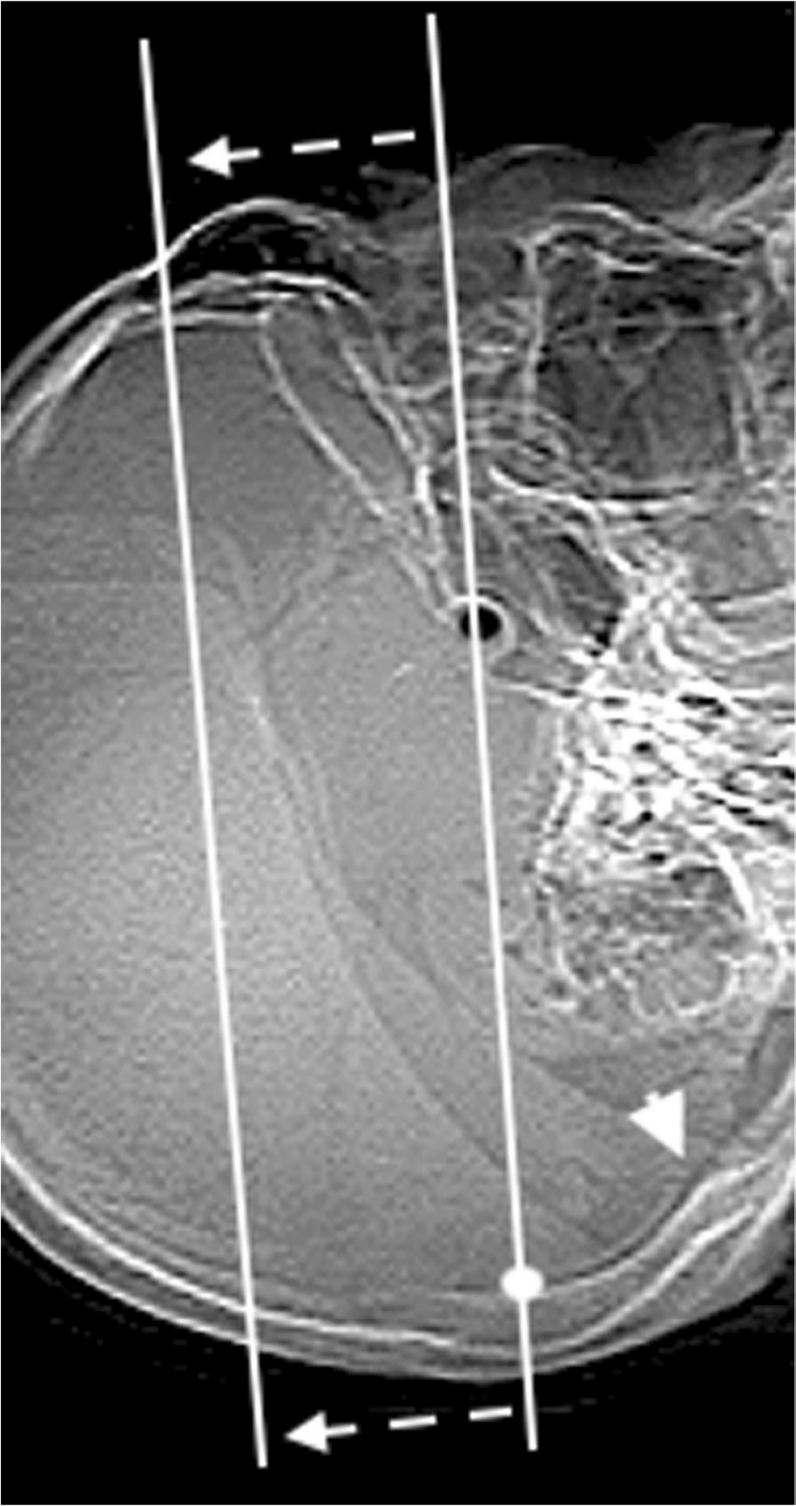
A CT scout image. The CT acquisition started from the line (inferior white line) connecting the pituitary fossa (black dot) with the point (white dot) 2 cm upward from the internal occipital protuberance (arrowhead) by tilting the gantry. CT scanning was performed with 4-cm coverage (dashed arrows and superior white line). CT, computed tomography
